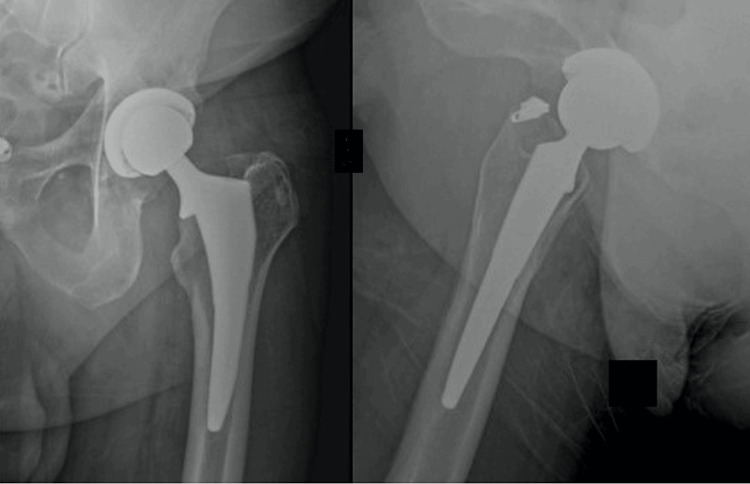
Anterior/posterior and lateral images of medially collared triple-tapered stem implanted via a direct anterior approach. X-ray images of medially collared triple-tapered (MCTT) stem at seven years post-implantation.
